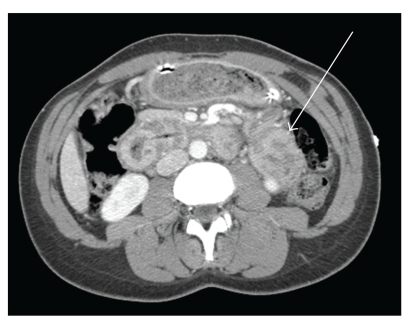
CT at initial presentation indicating intussusception of a short segment of small bowel.
